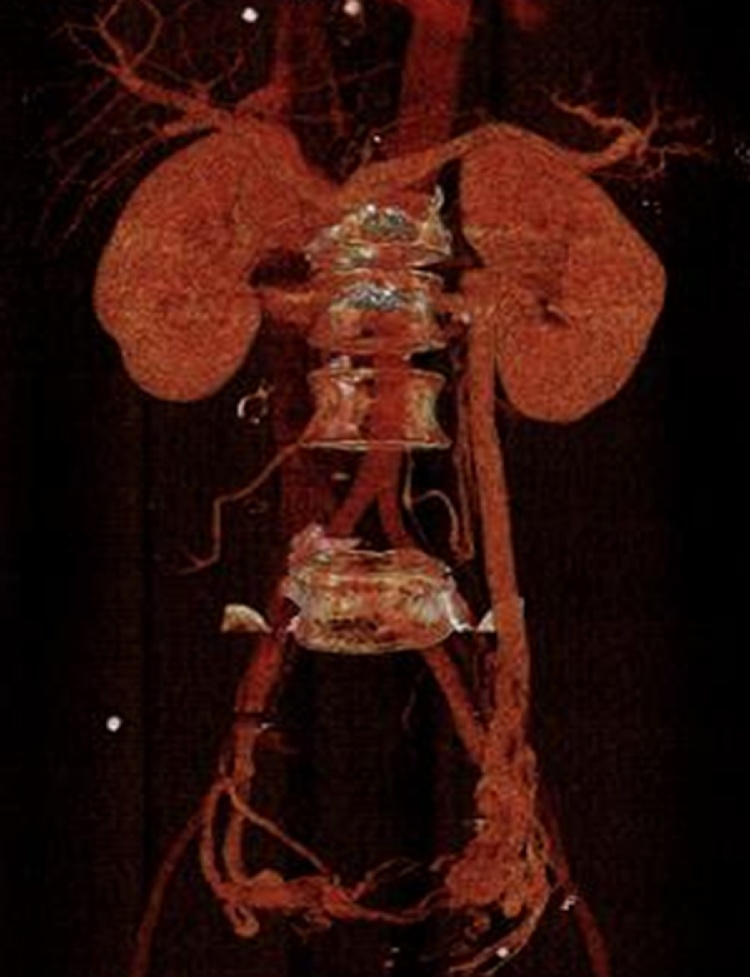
Follow-up CT scan, showing that drainage of the left renal vein was maintained through the ovarian vein.
